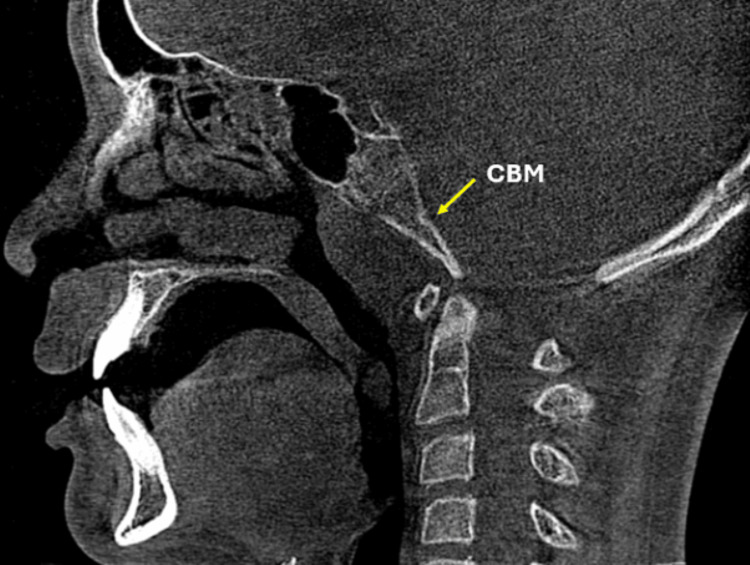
Sagittal CBCT section showing Canalis Basilaris Medianus (CBM) as a radiolucent depression in the basiocciput. CBCT, cone-beam computed tomography.
